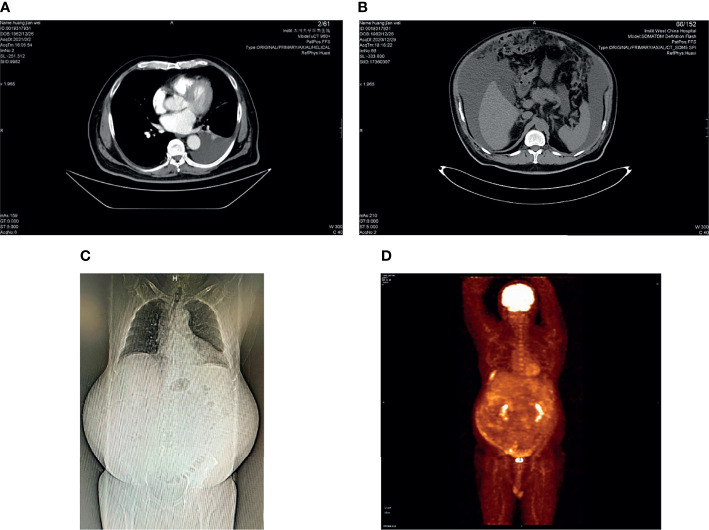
CT and PET-CT photos: (A) left pleural effusion, axial CT scan; (B) massive ascites, axial CT scan; (C) coronal CT scan; (D) PET-CT: there was inhomogeneous peritoneal thickening accompanied by abnormally increased glucose metabolism. We were unsure if this was a tumor or just inflammation.
